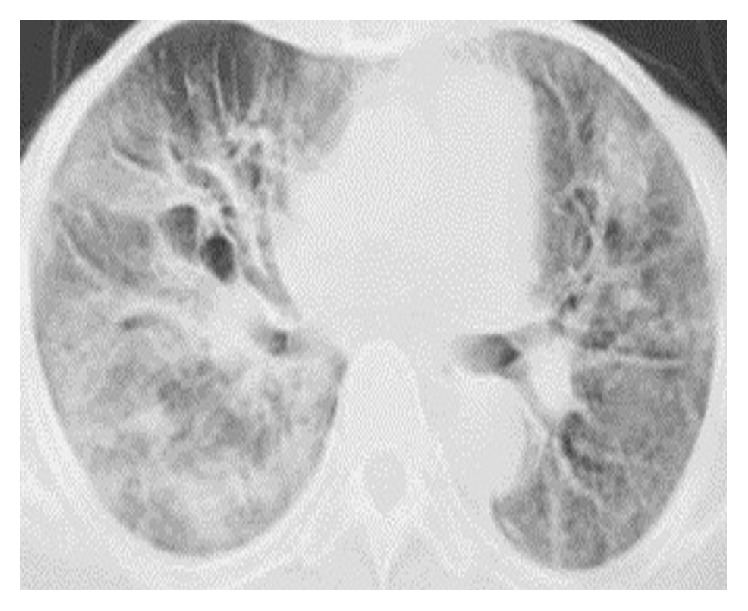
Section of the HRCT chest done on presentation showing bilateral ground glass opacities and a degree of bronchiectasis.
